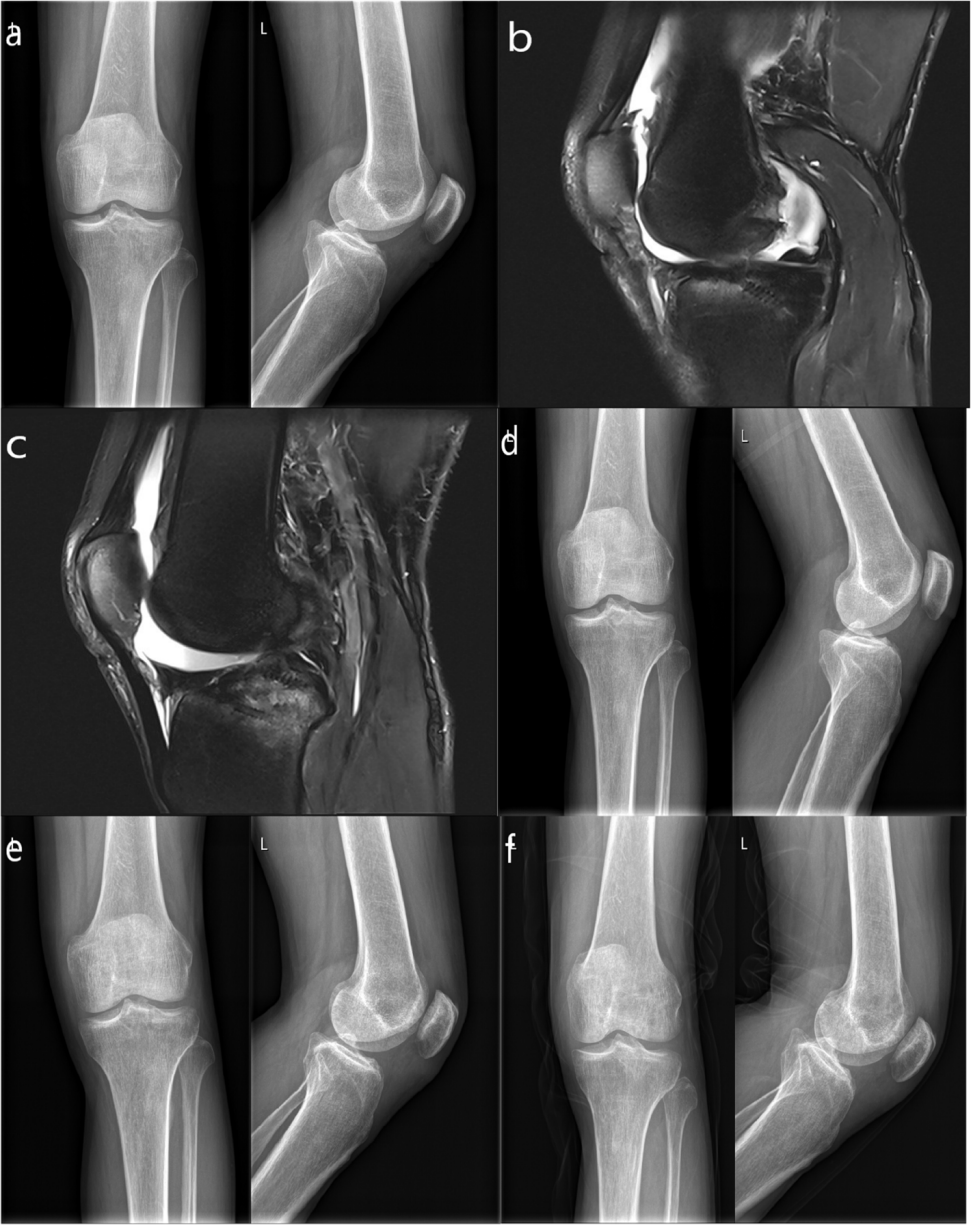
Postoperative images. a Postoperative radiographs showed a good reduction of displaced tibial eminence fracture fragment. b, c MRI image showed the restoration of the displaced fracture fragments and the “Dead Angle” of the suture anchor. d The radiograph showed good union of fracture at 3 months after operation. e The X-ray image showed excellent union at 6 months after operation. f Posterior and lateral radiograph showed a complete bony union at the final following-up
